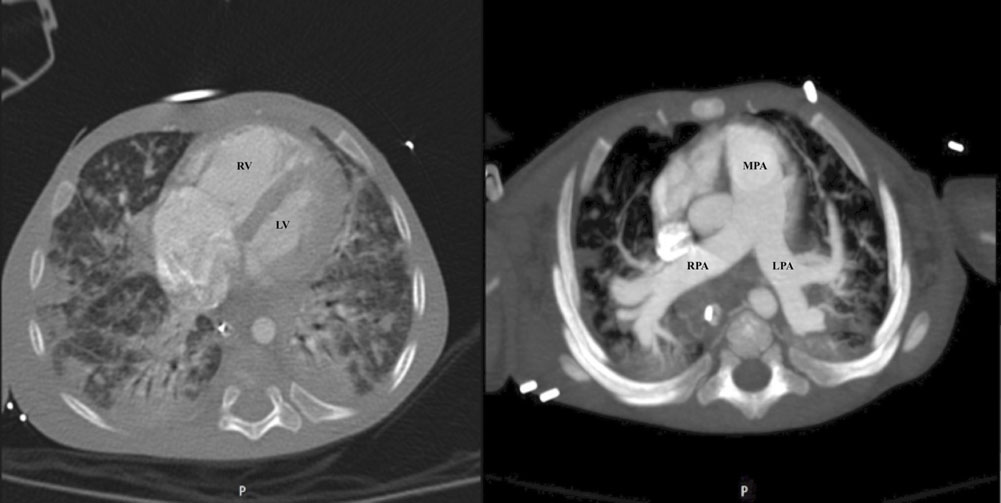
Thoracic angio-CT scan. The left panel shows multifocal regions of consolidation and ground-glass opacifications with peripheral and basal predominance. The right ventricle is severely dilated. No pleural or pericardial effusion. The right panel shows a severely dilated pulmonary artery with no signs of pulmonary thromboembolism (Maximum intensity projection reconstruction).RV (right ventricle); LV (Left ventricle); MPA (main pulmonary artery); LPA (left pulmonary artery); RPA (right pulmonary artery).
